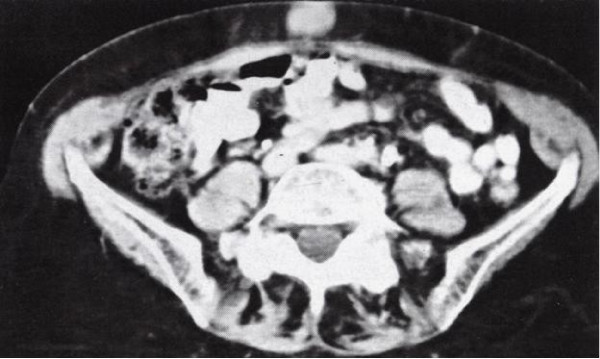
CT-scan of the abdomen: This image shows an oval umbilical mass measured 2 cm.
